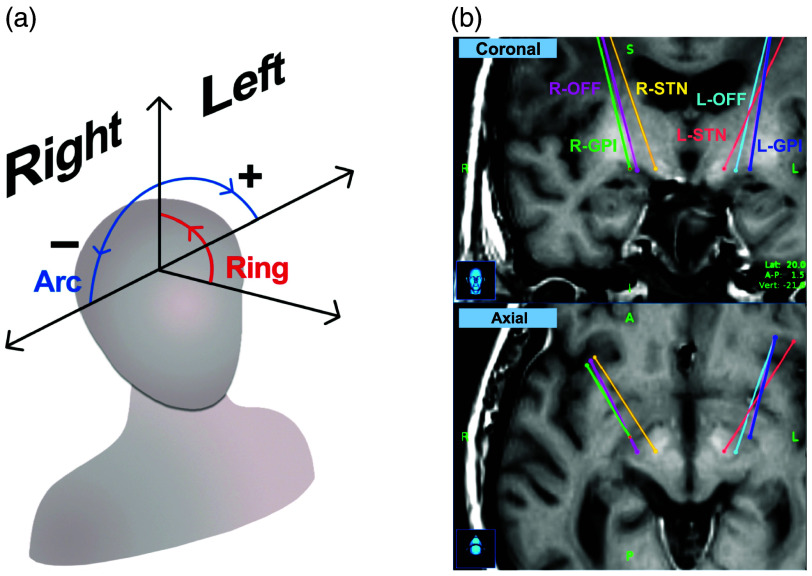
Trajectory planning prior to the DBS surgery. (a) Coordinate system used for the insertion planning. The arc angle is positive for the left hemisphere and negative for the right hemisphere. (b) Insertion angles determined with the Medtronic StealthStation™ neuronavigation system using the preoperative MRI scans for the six trajectories.
